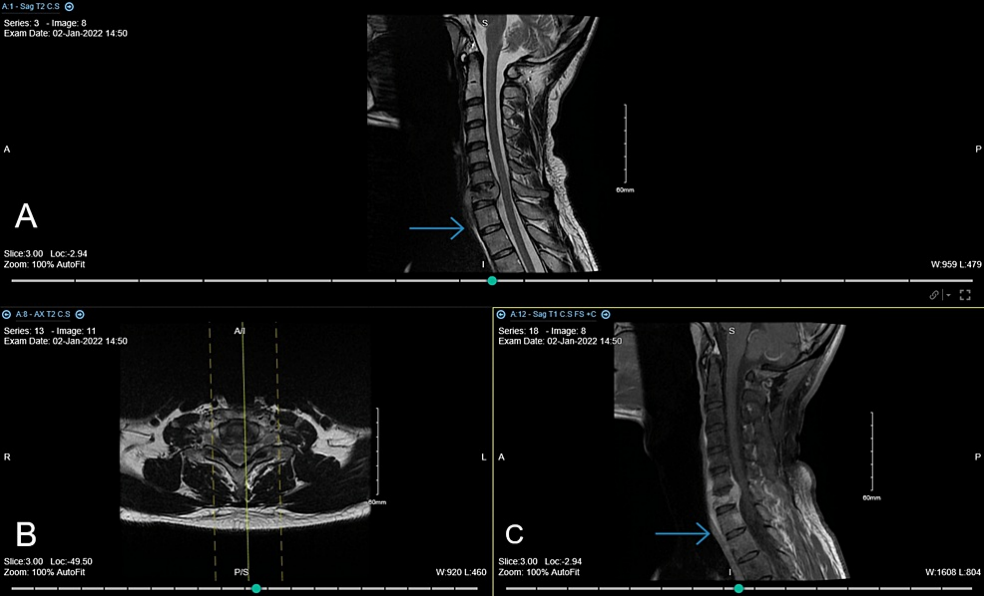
Cervical MRI brought by the patient to the first follow up showed progression of the destruction. Note regression of the retropharyngeal collection size to inferior border of T1. (A) T2 sagittal view, (B) axial view, and (C) T1 sagittal view.
